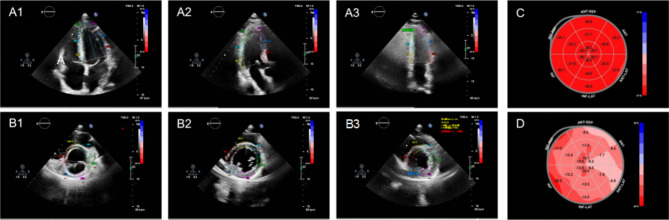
Analysis of layer-specific longitudinal and circumferential strain. The layer-specific longitudinal strain was derived from the apical 4-chamber view (A1), 3-chamber view (A2), and 2-chamber view (A3). The layer-specific circumferential strain was derived from the basal short-axis view (B1), midventricular short-axis view (B2), and apical short-axis view (B3). (C) Epicardial myocardial circumferential strain of a health. (D) Epicardial myocardial circumferential strain of a septic patient
